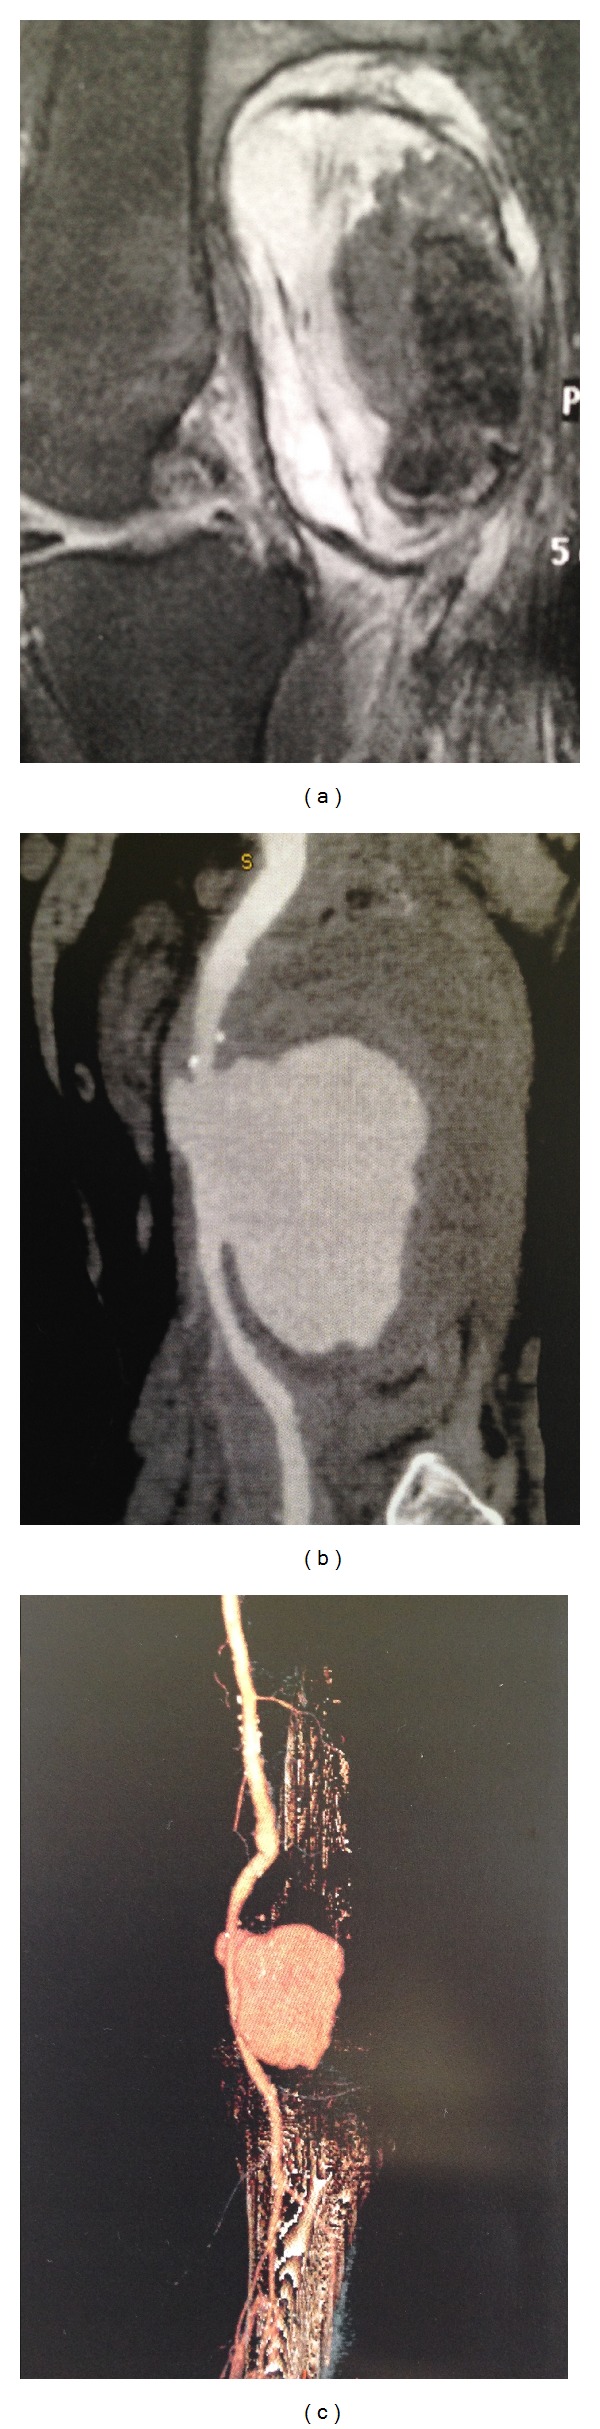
(a) Magnetic resonance imaging (MRI) showing the huge popliteal artery aneurysm. (b) Computed tomography angiography (CTA) depicting the huge aneurysm with significant thrombus inside the aneurysm sac. (c) 3D reconstruction of CTA showing the aneurysm with the inflow and outflow vessels.
